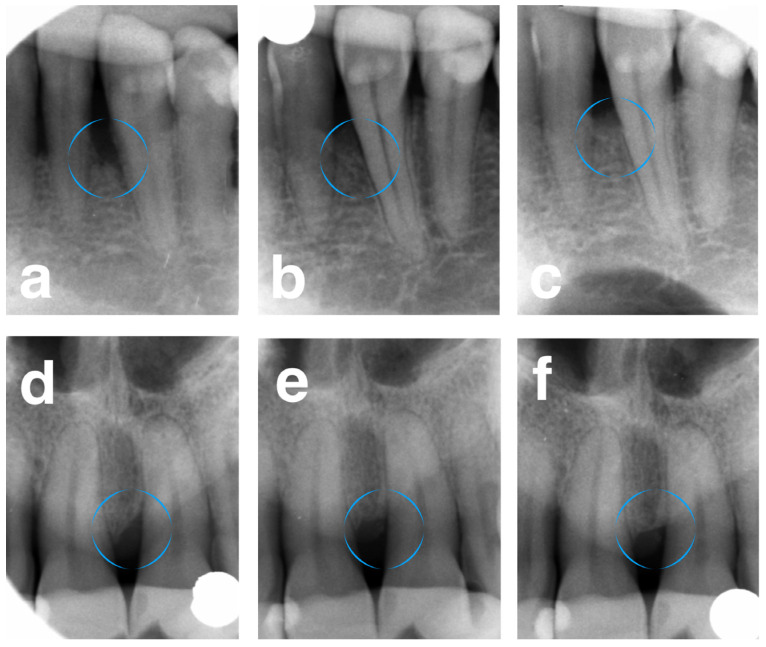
(a) Pre-operative X-ray of the infrabony defect on the mesial surface of tooth 33 (test site). (b) X-ray 1 year post-operatively. (c) X-ray 5 years post-operatively. (d) Pre-operative X-ray of the infrabony defect on the mesial surface of tooth 21 (control site). (e) X-ray 1 year post-operatively. (f) X-ray 5 years post-operatively. Circular outlines highlight infrabony defects.
